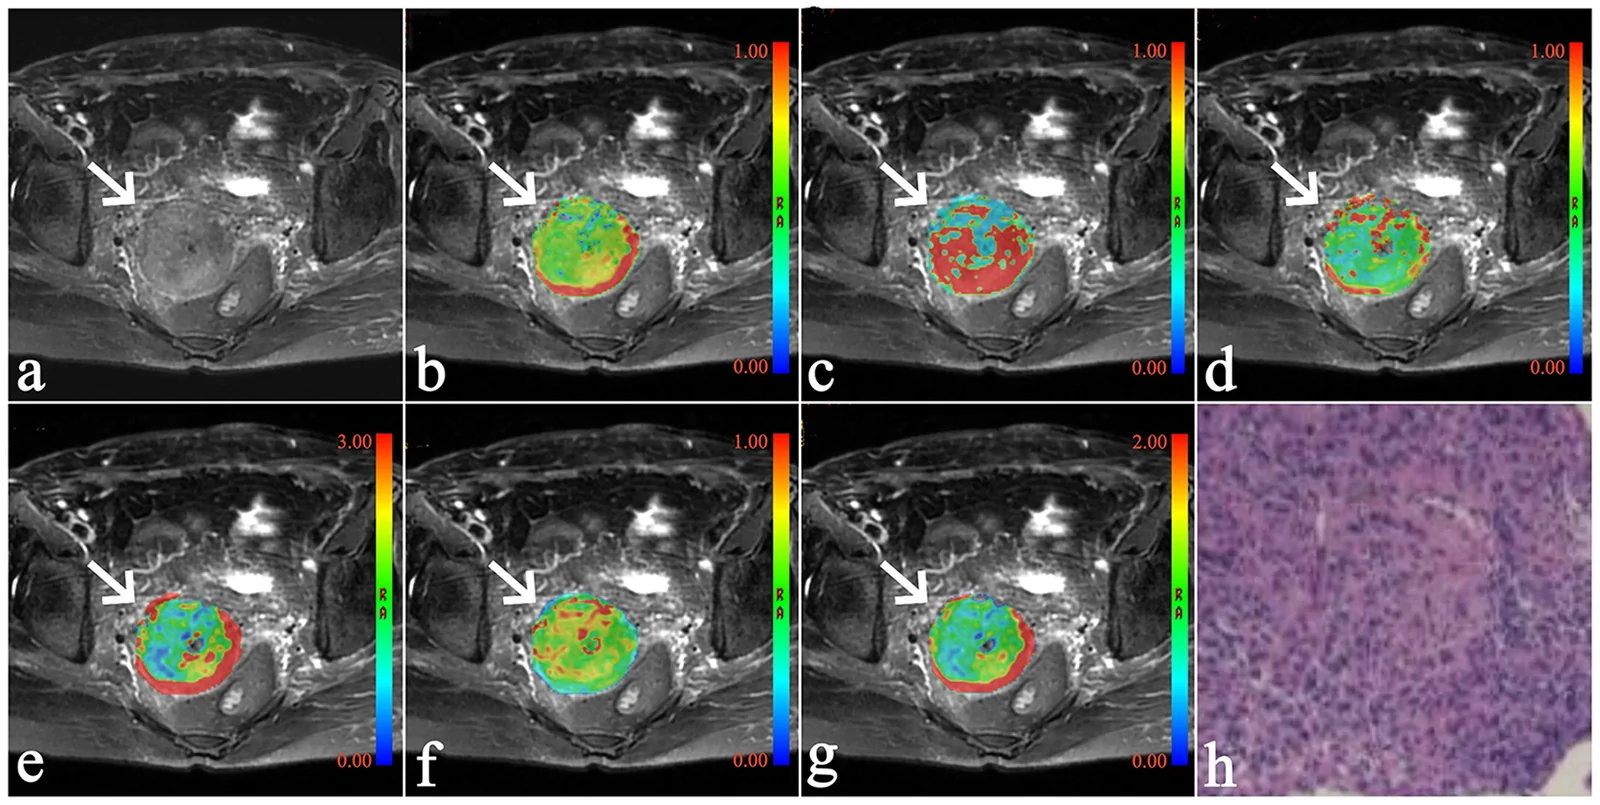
A 75-year-old woman with cervical cancer (Ki-67 = 70%). (a) Map of T2-weighted imaging; (b) Pseudo colored map of *f*_1_; (c) Pseudo colored map of *f*_2_; (d) Pseudo colored map of *f*_3_; (e) Pseudo colored map of MD; (f) Pseudo colored map of MK; (g) Pseudo colored map of ADC; (h) Pathological images (original magnification, × 100).
